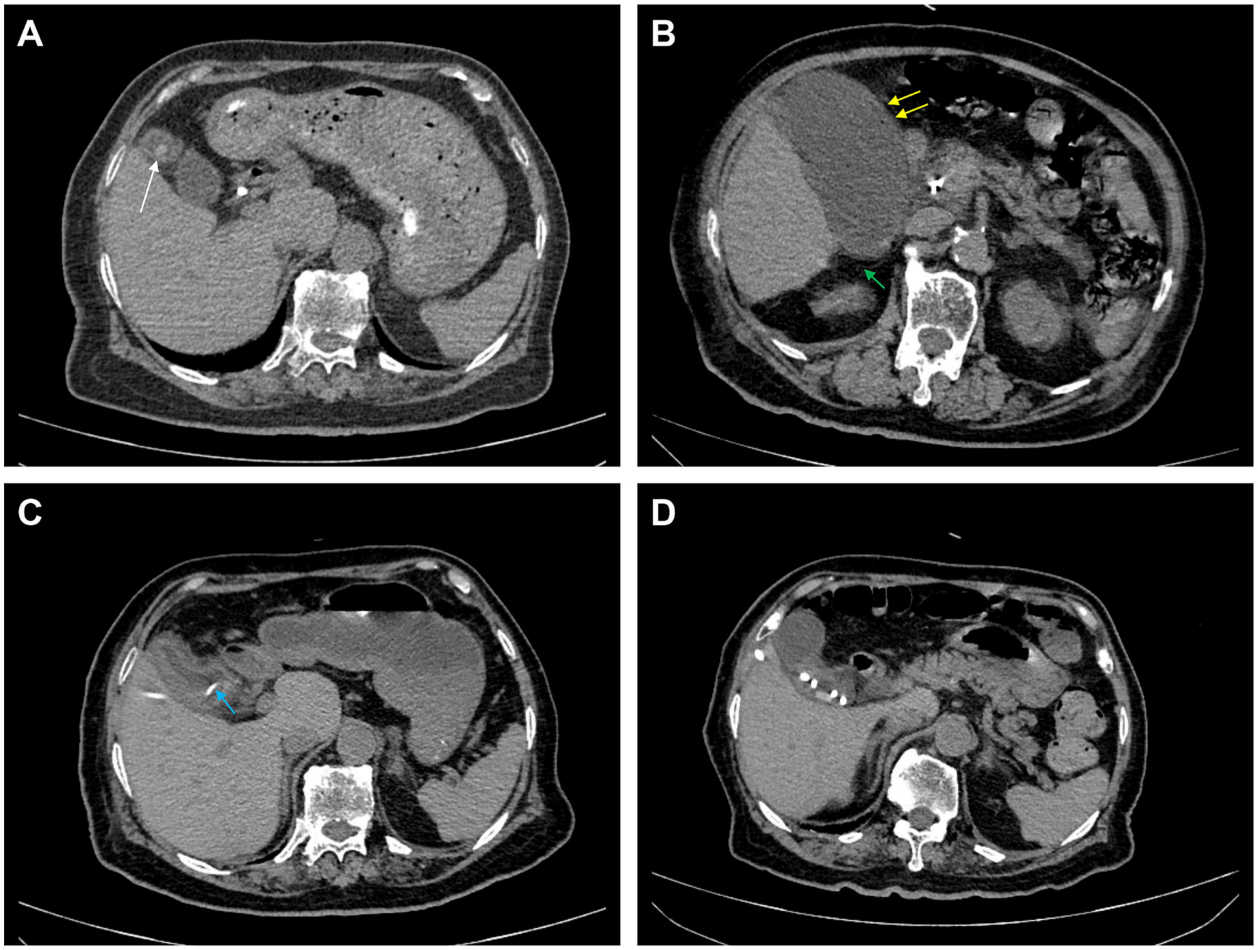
Abdominal CT scan of the patient post admission. (A) Baseline (3 months before admission): CT shows gallstones (white arrow) with a normal gallbladder and no inflammation. (B) Acute phase (4 days post admission): CT reveals a swollen gallbladder with wall thickening (yellow arrowhead) and significant surrounding stranding (green arrow). (C) Post-drainage (9 days post admission): CT after PTGBD shows a decompressed gallbladder with a drainage catheter (blue arrow). (D) One-month follow-up: Gallbladder remains contracted and thickened.
